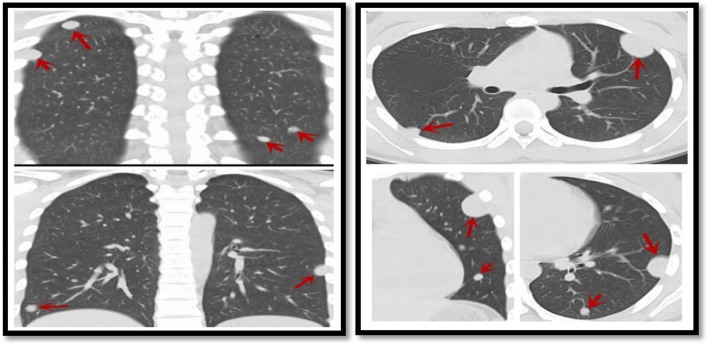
Chest CT (Multiple spindle cell hemangiomas in both lungs)
